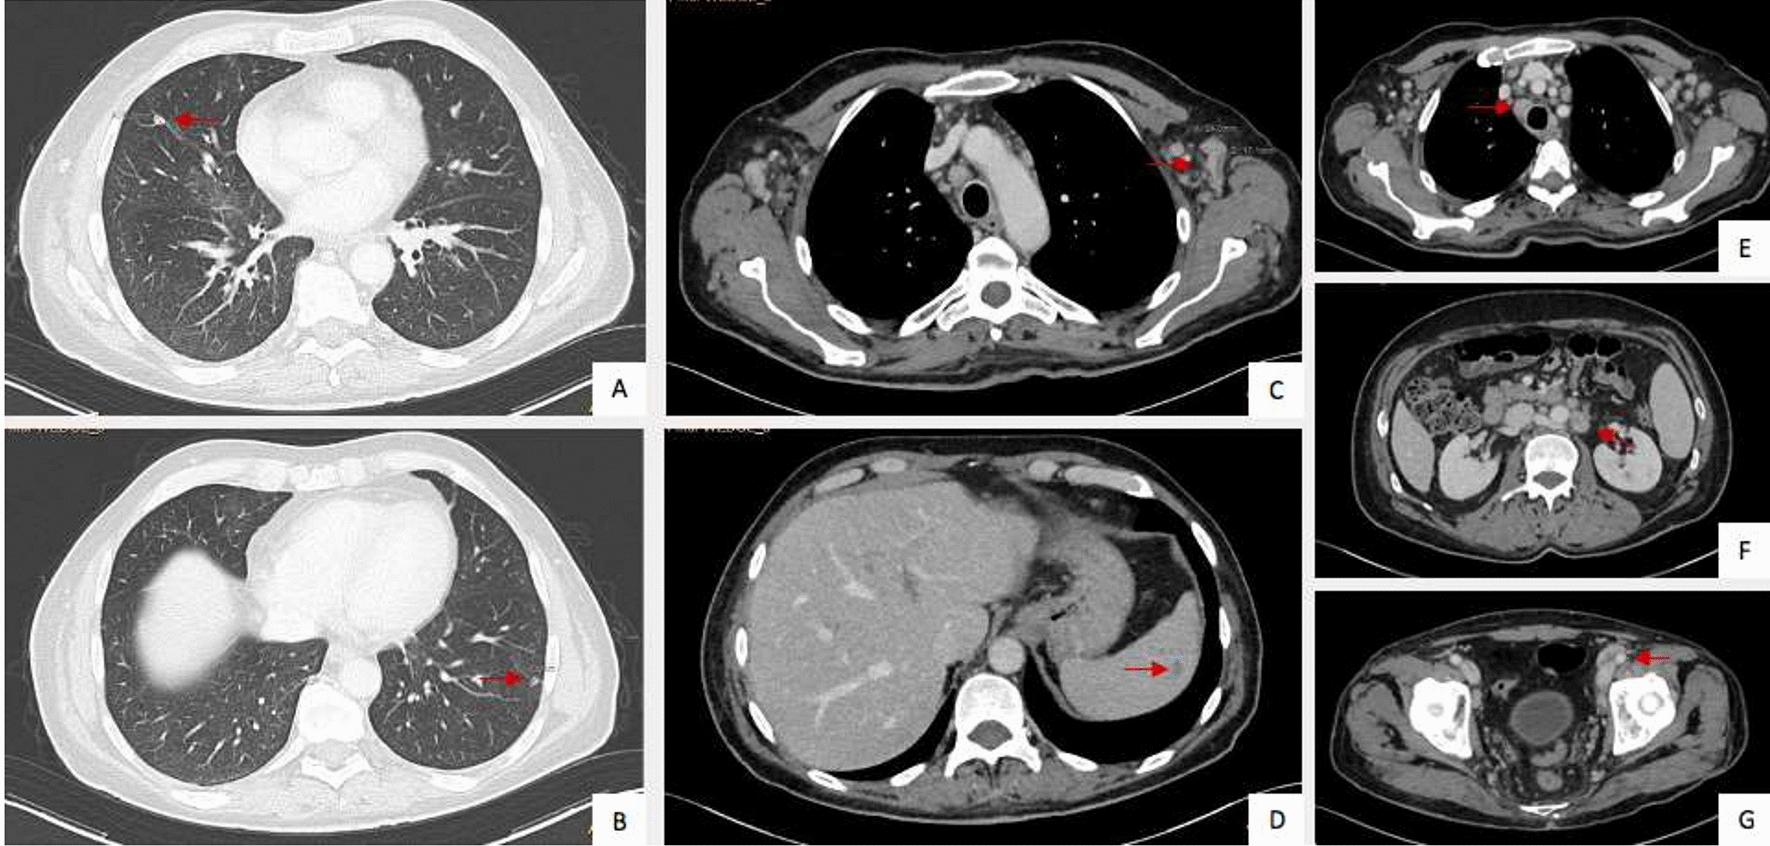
Disseminated Kaposi sarcoma revealed by the total-body CT scan. Courtesy of University Radiology Division, Spedali Civili Hospital, Brescia (Italy). CT scan images show lung parenchymal nodules (A, B), axillary lymph adenomegalies (C), splenic lesion (D), pre-tracheal (E), retroperitoneal (F), and inguinal (G) lymph adenomegalies
